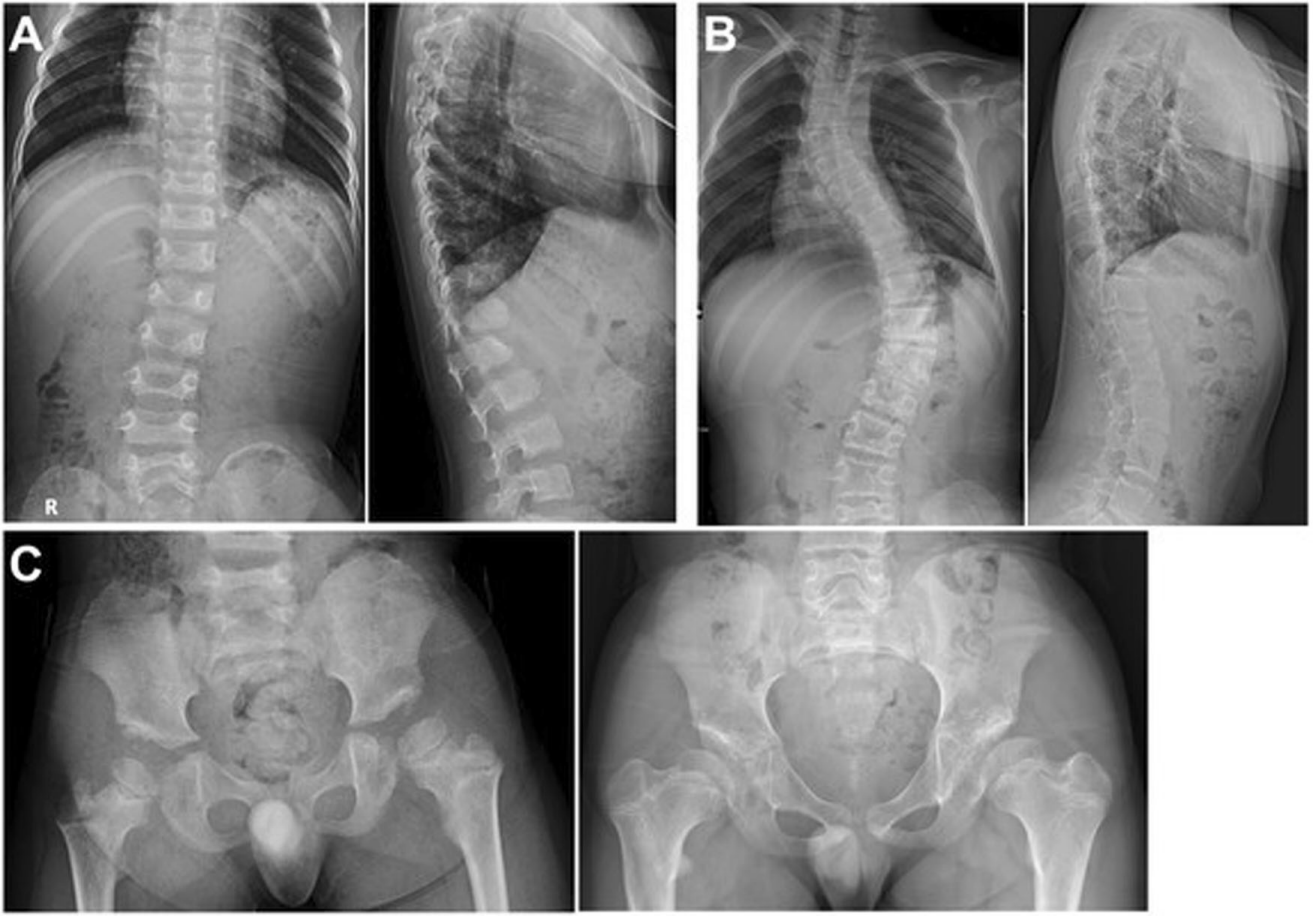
Radiographs of the spine and hip. A Radiographs of the spine at 5 years of age showing mild scoliosis and L1 dysplasia with anteroinferior beaking. B Radiographs of the spine at 11 years of age showing progressed thoracolumbar scoliosis. C Hip radiograph at 5 years of age (left) showing hip dysplasia with a flat and irregular acetabular roof and at 11 years (right) showing a steep acetabular roof. The proximal femur had coxa breva and coxa vara deformities. The femoral head was poorly covered by the acetabulum but not dislocated.
